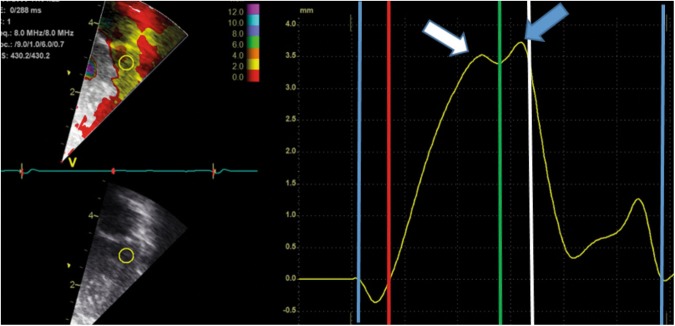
Same color-coded tissue Doppler velocity recording as in upper panel of Fig. 2, the curve now shows the displacement of the sample area. White arrow at the peak systolic displacement. Blue arrow at the peak displacement, occurring early in diastole in this image. Peak (global) displacement can also occur in systole. Blue vertical lines denote closure of the mitral valve. Red vertical line denotes opening of the aortic valve. Green vertical line denotes closure of the aortic valve. White vertical line denotes opening of the mitral valve. *X*-axis: time. *Y*-axis: displacement assessed by velocities from the sample area
